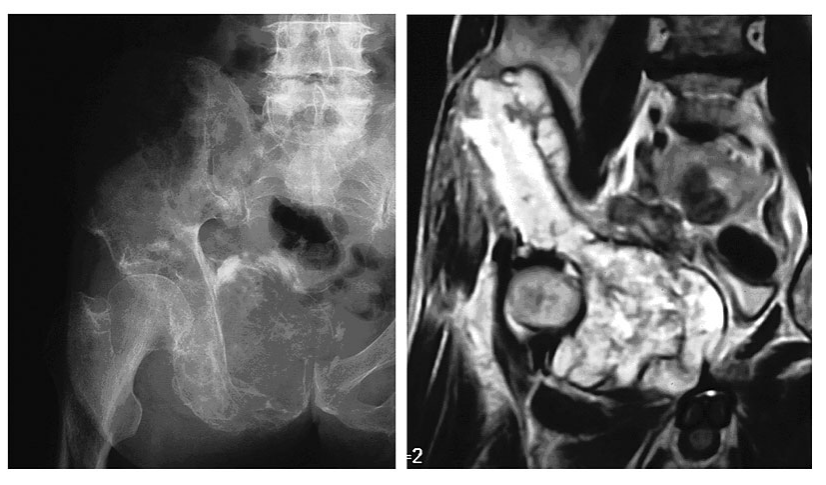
Roentgenographs show an expansive lesion with punctate calcification in the right pelvic bone (left). MRI shows a lobulated lesion, protuberant to the pelvic cavity, in the pelvic bone with high-intensity on T2-weighted image (right).
